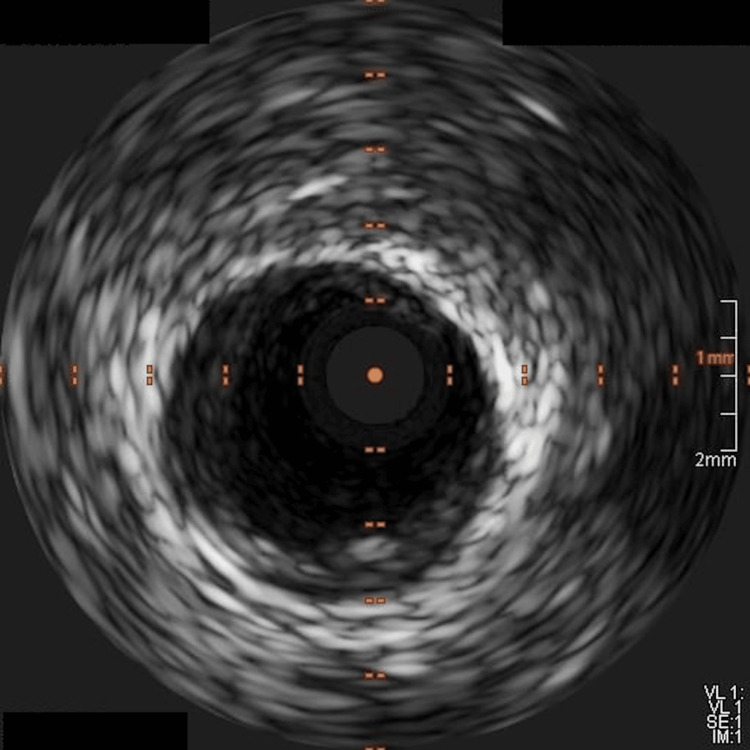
Intravascular ultrasound (IVUS) of the proximal right coronary artery after intracoronary nitroglycerin administration demonstrating minimal plaque burden and no thrombus, supporting coronary vasospasm rather than a fixed atherosclerotic lesion.
